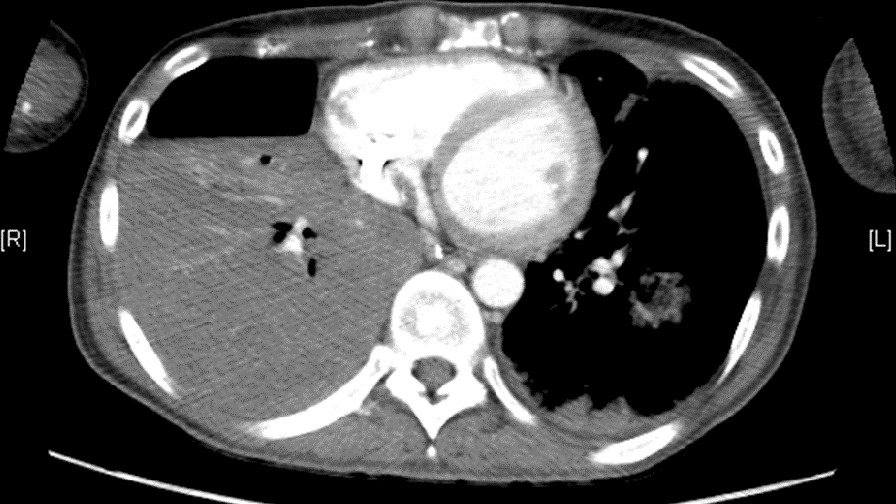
Preoperative computed tomography reveals pneumorrhagia in the right lung
